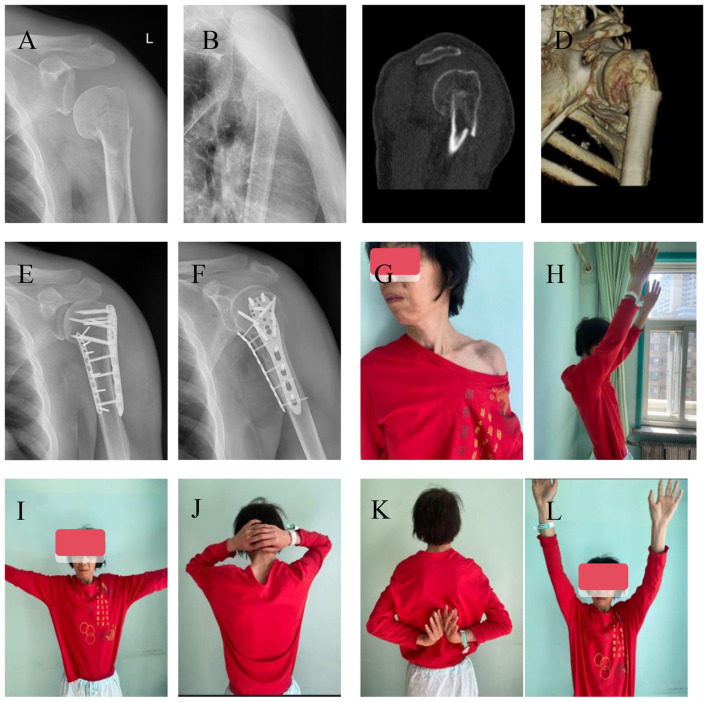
A 65-year-old female suffered a proximal humeral fracture due to a traffic accident with loss of the medial humeral tuberosity support. She was treated with double plate technique. The patient was followed up for 16.2 months. (A, B) X-ray anteroposterior and lateral views showed loss of the medial column support of the proximal humerus and varus deformity of the humeral head. (C, D) Sagittal and three-dimensional CT reconstruction showed loss of the medial column of the humeral tuberosity. (E, F) The last follow-up X-ray anteroposterior and lateral views showed anatomical reduction of the fracture, no displacement of the internal fixation, and fracture healing. (G–L) The appearance of the left shoulder after surgery and the recovery of shoulder joint flexion, abduction, external rotation and extension functions at the last follow-up were good.
